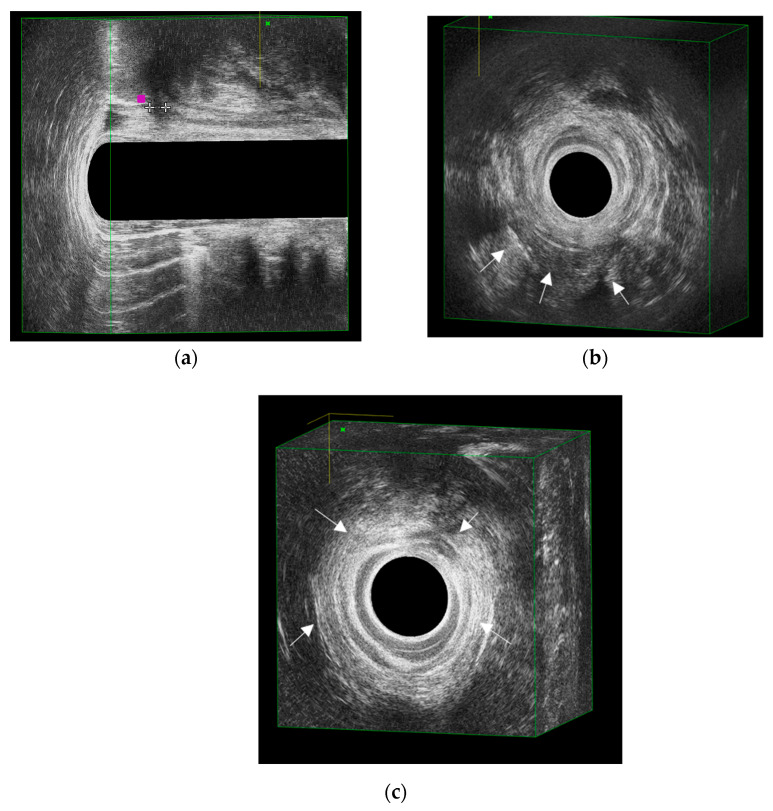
Representative EAUS features of Crohn’s-related perianal fistulas. (a) Tract width > 4 mm, visualized as a hypoechoic tubular structure exceeding the normal diameter of cryptoglandular tracts; (b) Crohn’s Ultrasound Fistula Sign (CUFS), defined as a hypoechoic tract surrounded by a thin hypoechoic rim and an outer hyperechoic halo; (c) Rosary sign, characterized by a discontinuous “rosary bead”-shaped hypoechoic halo in the intersphincteric space of the upper anal canal.
